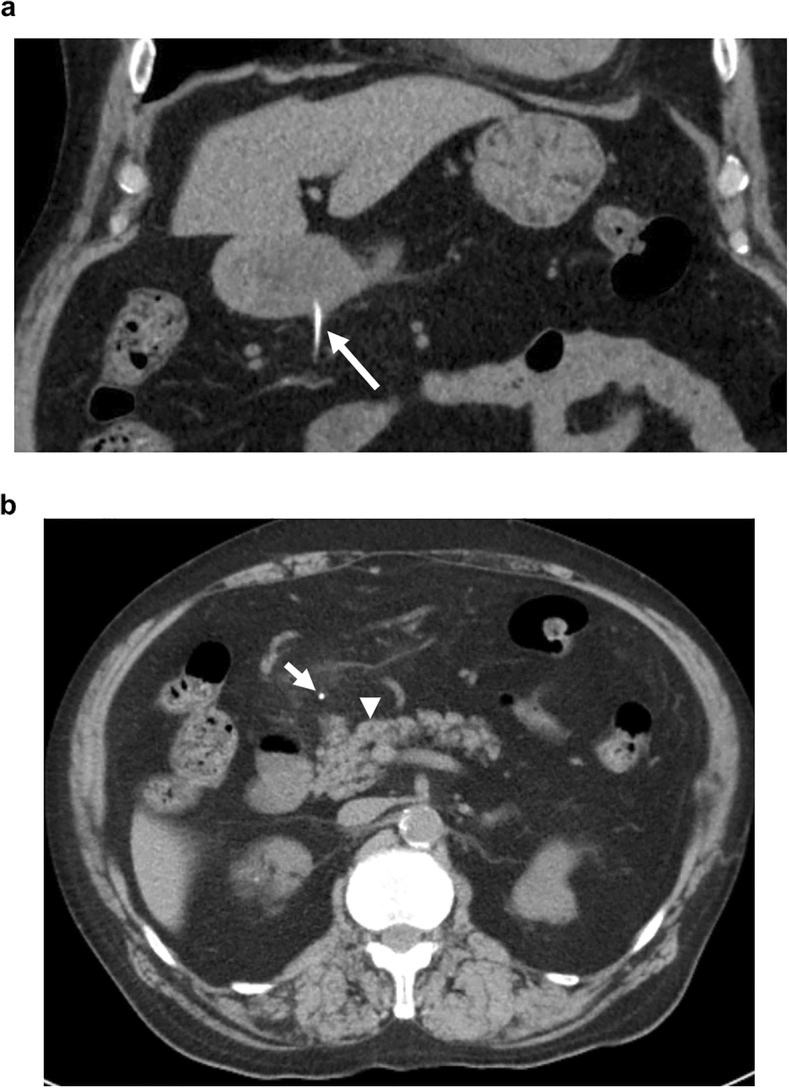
Coronal view of a computed tomography (CT) image showing a linear, hyperdense, foreign body (arrow), which appeared to penetrate through the posterior wall of the gastric antrum (a). CT image showing the foreign body (arrow) and the pancreas (arrowhead) (b)
